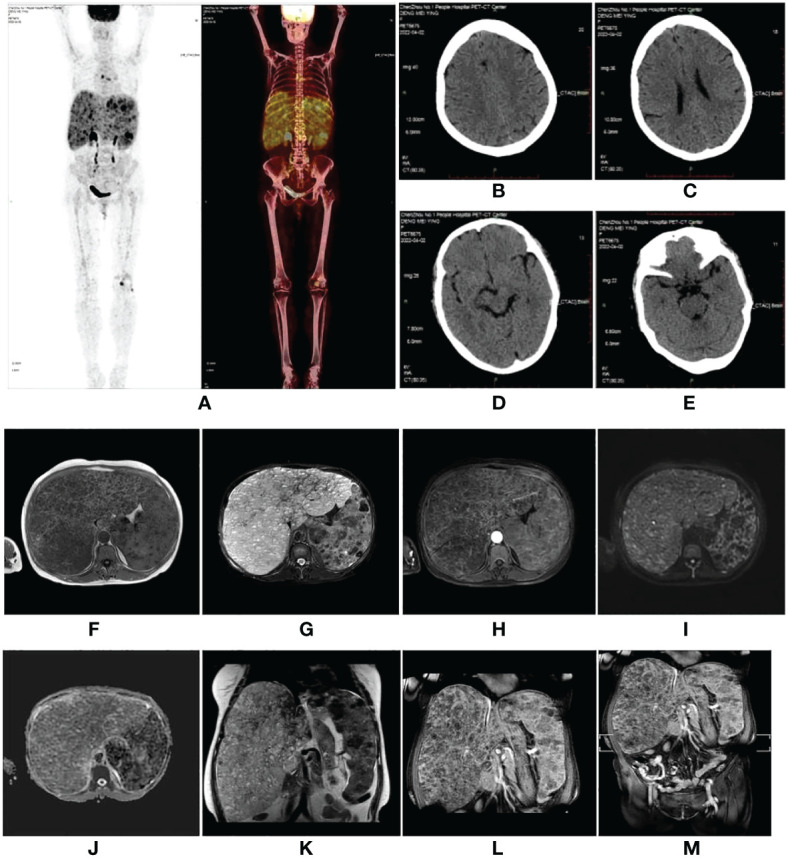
(A–E) Fluorodeoxyglucose positron emission tomography (FDG-PET) scan performed at the local hospital. The images showed diffuse hepatomegaly and splenomegaly with a slightly increased FDG uptake, without other abnormal FDG accumulation or intracranial lesions. (F–M) A magnetic resonance imaging scan of the abdomen revealed hepatosplenomegaly with countless nodules of various sizes involving the whole liver and spleen, which showed hypointensity on T1-weighted (T1W) images (F), hyperintensity on T2-weighted (T2W) images (G), and inhomogeneous enhancement in diffusion MRI (I, H, L). Diffusion-weighted imaging (DWI) (I) showed a slightly higher signal intensity; however, the apparent diffusion coefficient (ADC) (J) value did not decrease. (F) T1W image. (G) Fat-saturated T2-weighted sequence (fsT2W). (H) The enhanced scan in the transverse view. (I) DWI. (J) ADC. (K) T2W image in the coronal view. (L) The enhanced scan in coronal view. (M) The enhanced scan of the whole abdomen in coronal view.
